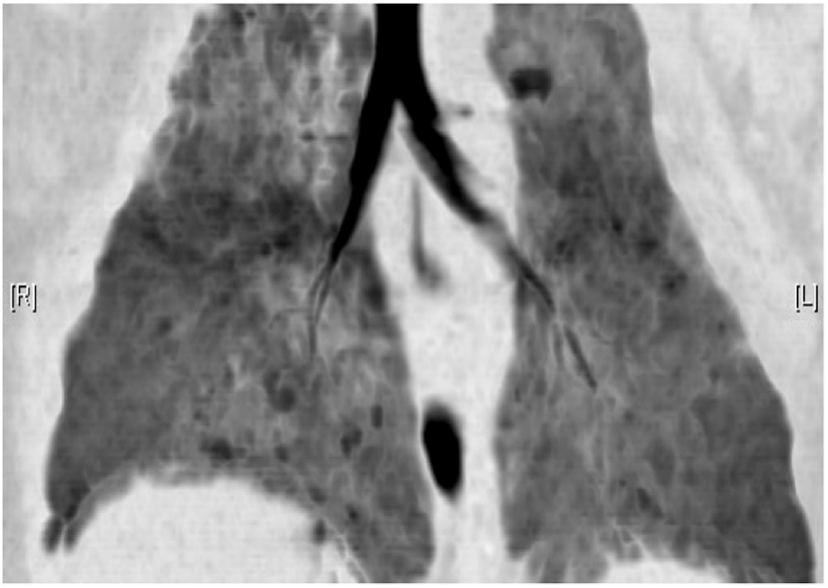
Minimum intensity projection CT reconstruction demonstrating airway
